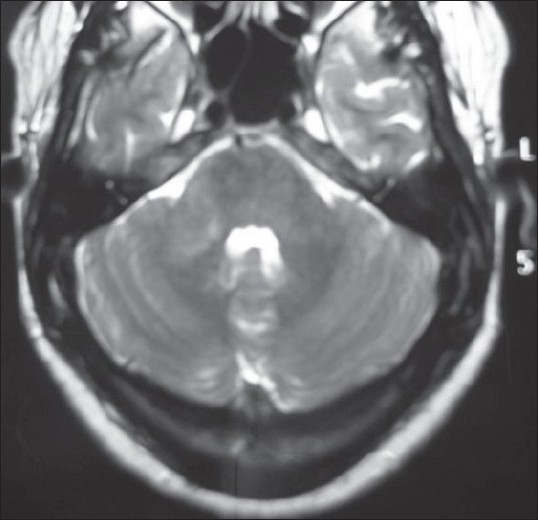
MRI (T2 axial) done 4 weeks after presentation showing resolution of the lesions
